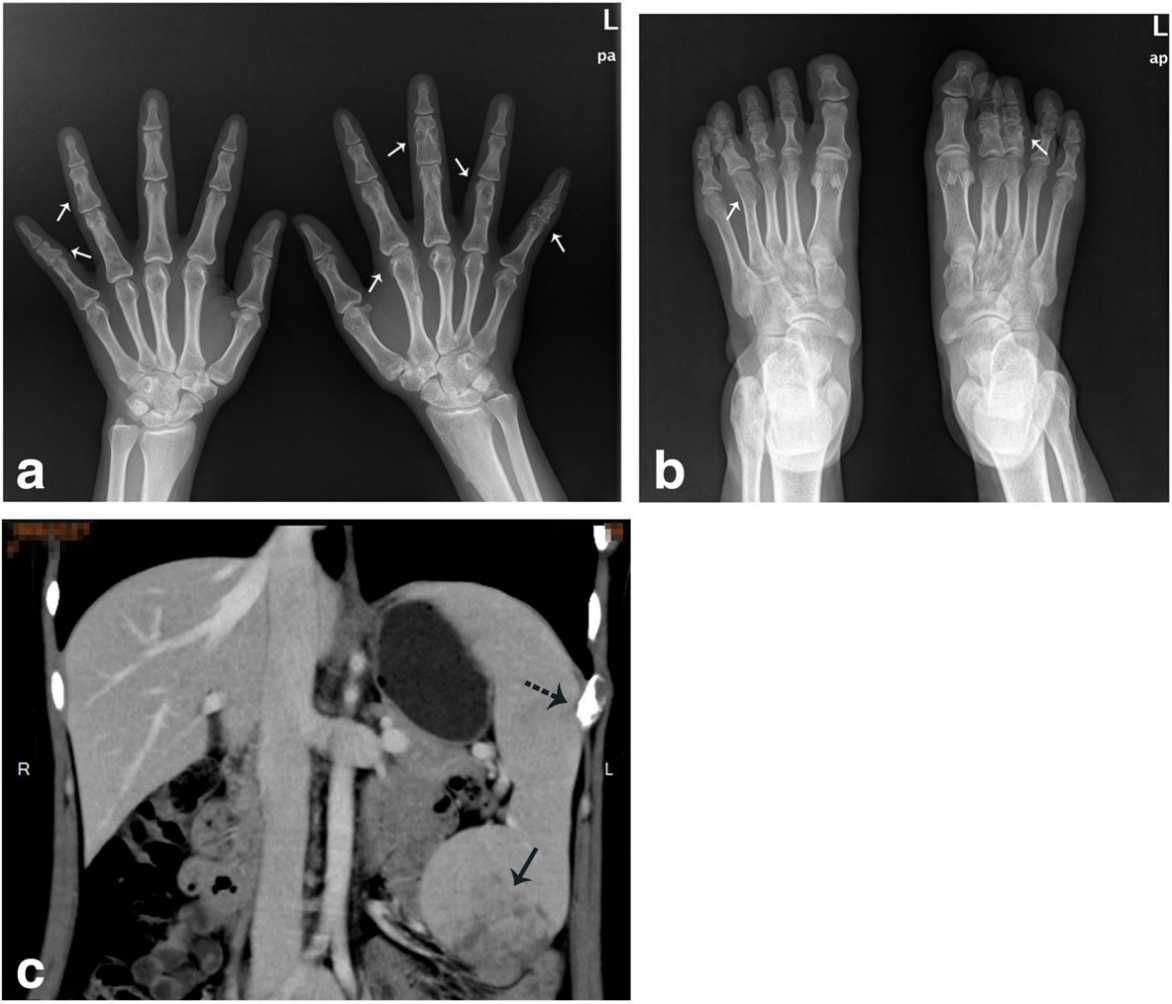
Preoperative imaging studies. CR images of both hands (a) and feet (b) show multiple bone deformations (white arrows). The abdominal CT (c) demonstrates a huge nodular hyperplasia of the spleen which is considered as angioma (black solid arrow), and a vascular lesion of the 10th rib (black doted arrow)
